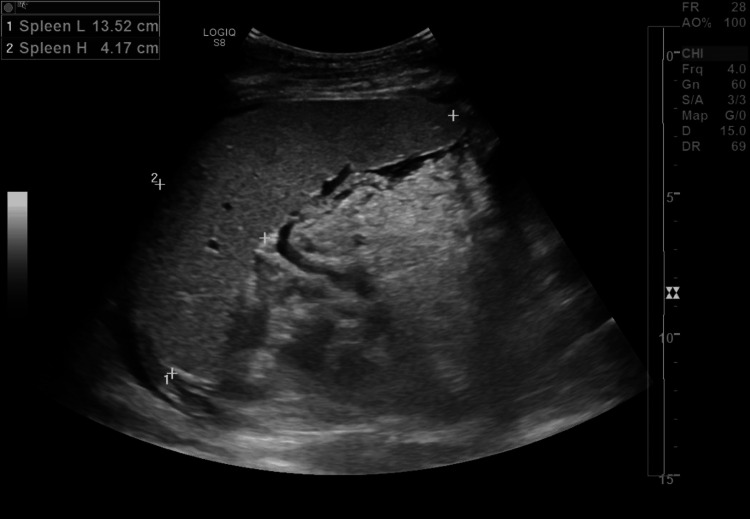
Abdominal ultrasound Spleen at the upper limit of normal size
